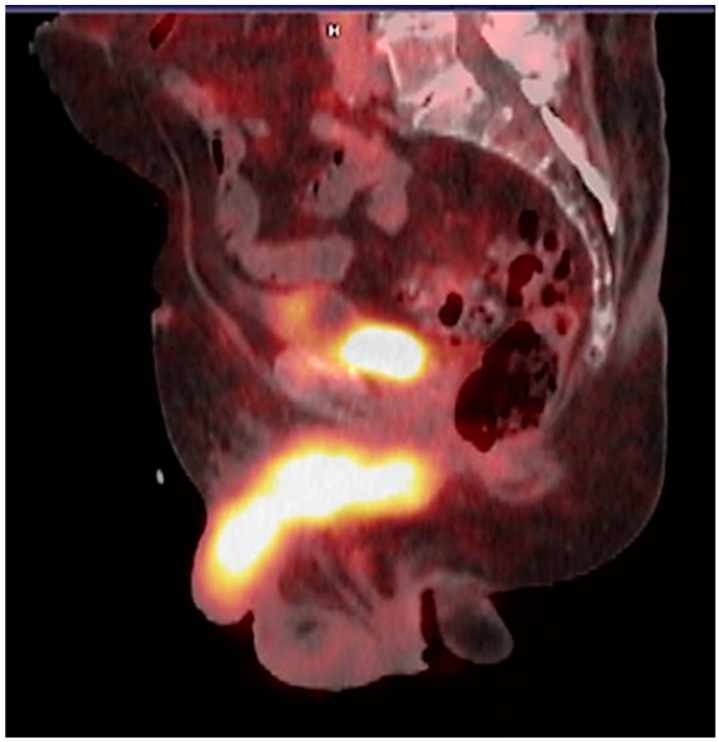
PSMA PET/CT demonstrated an unusual sight of metastasis infiltrating the penis of a patient with prostate adenocarcinoma.
